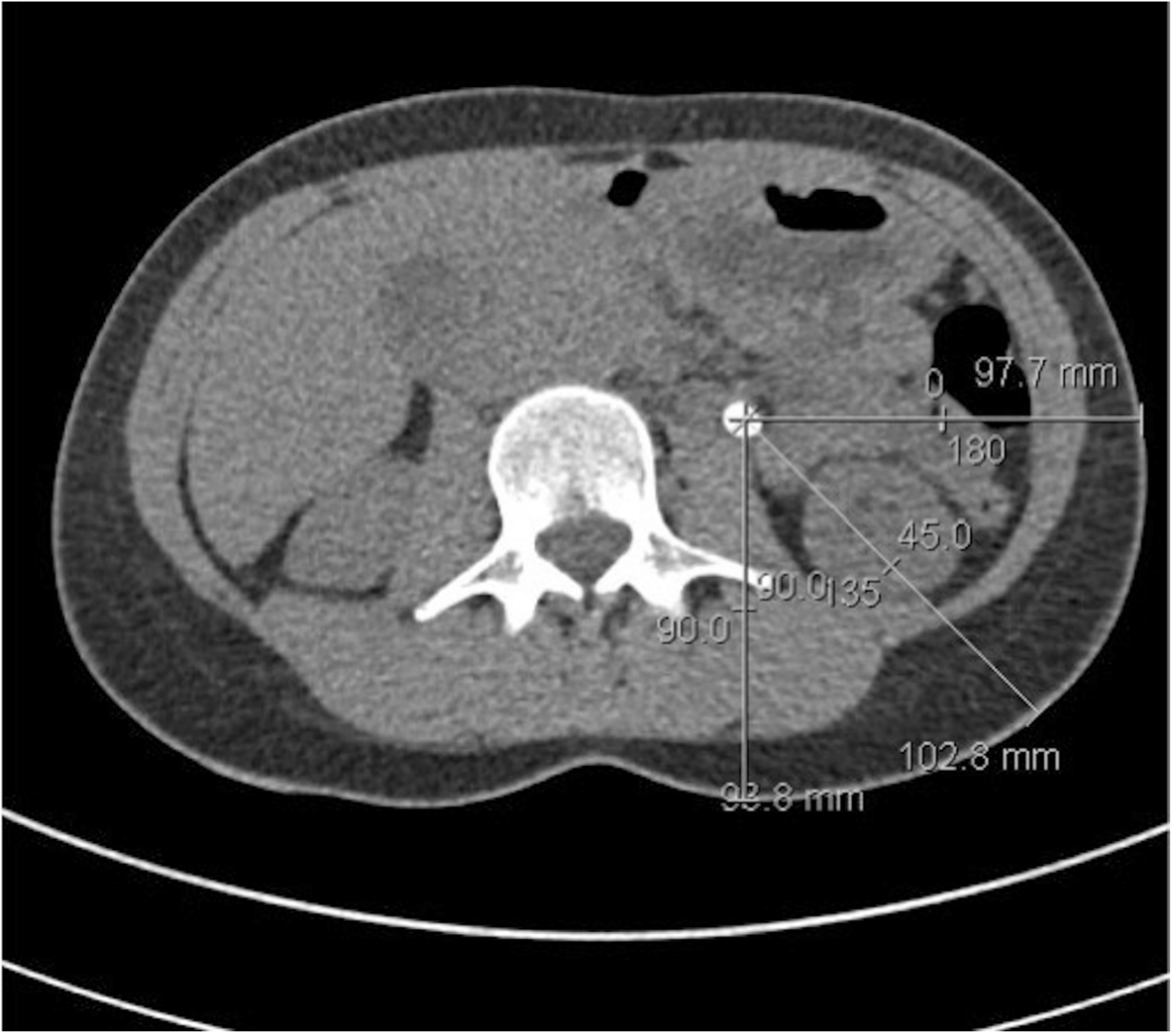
Skin-to-stone distance (SSD) was calculated by the measuring distances from Stone-to-skin at 0°, 45°, and 90° using radiographic calipers
